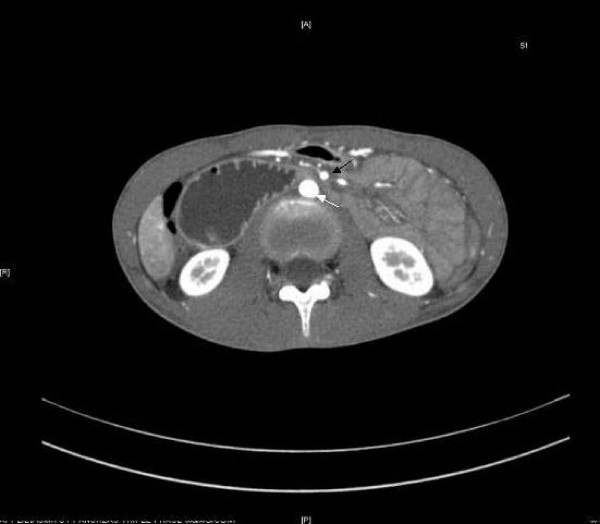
Computed tomography scan demonstrating compression of the duodenum between the patient's aorta (white arrow) and superior mesenteric artery (black arrow).
